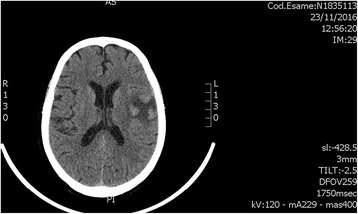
Ischemic hypodensity involving the left Sylvian fissure on brain computed tomography
